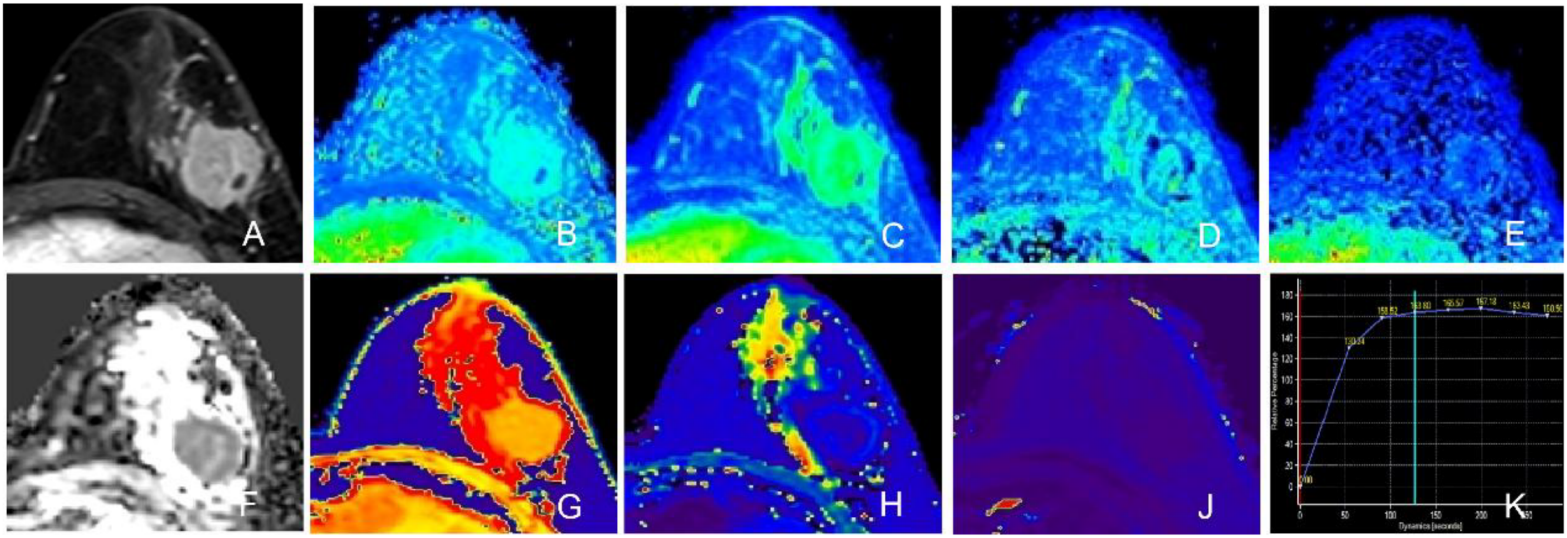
Representative images of a non-TNBC lesion (Luminal B subtype, Invasive Ductal Carcinoma, Ki-67: 30%) in the left breast. The tumor exhibits an irregular shape with spiculation and heterogeneous enhancement. (A) Axial DCE-MRI image. (B) Relative Enhancement (RE) map. (C) Maximum Enhancement (ME) map. (D) Wash-in rate (WI) map. (E) Wash-out rate (WO) map. (F) ADC map. (G) Native T1 map. (H) Enhanced T1 map. (J) T2 map. (K) Time-Intensity Curve (TIC) showing a plateau pattern (Type II).
